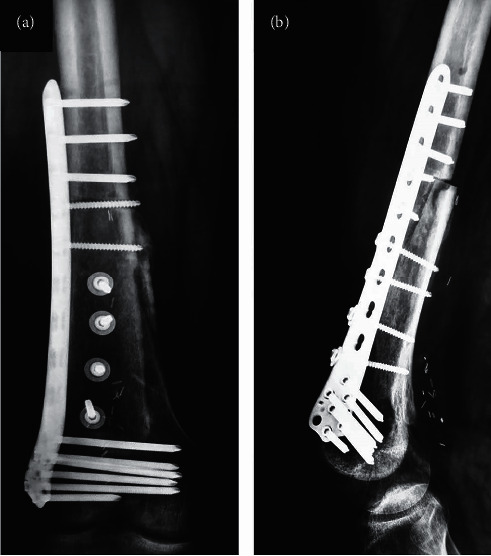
Anteroposterior (a) and lateral radiographs (b) nine months postoperatively showing incorporation of the allograft at the distal part. However, the proximal part of the allograft shows partial union.
